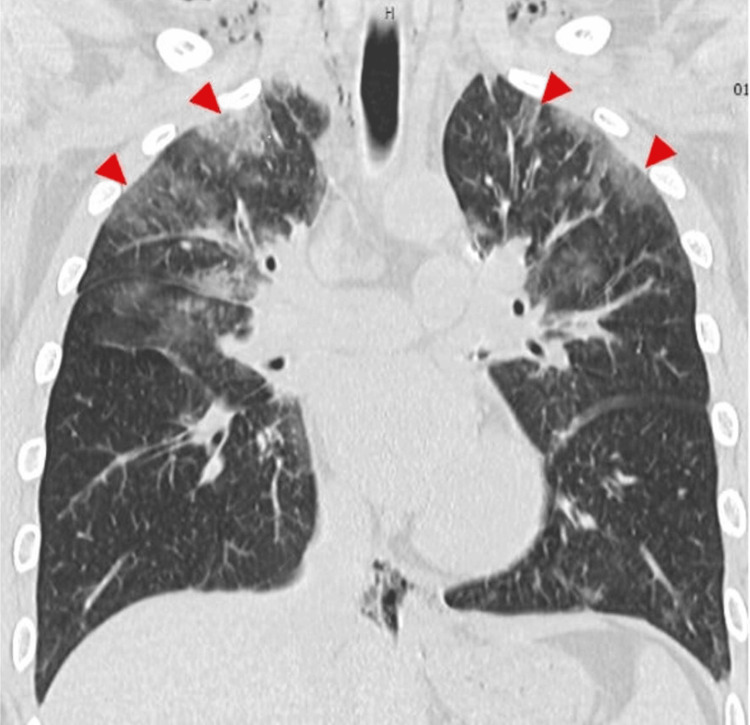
Contrast-enhanced CT showed bilateral apical infiltrates without abscess formation (red arrows).
